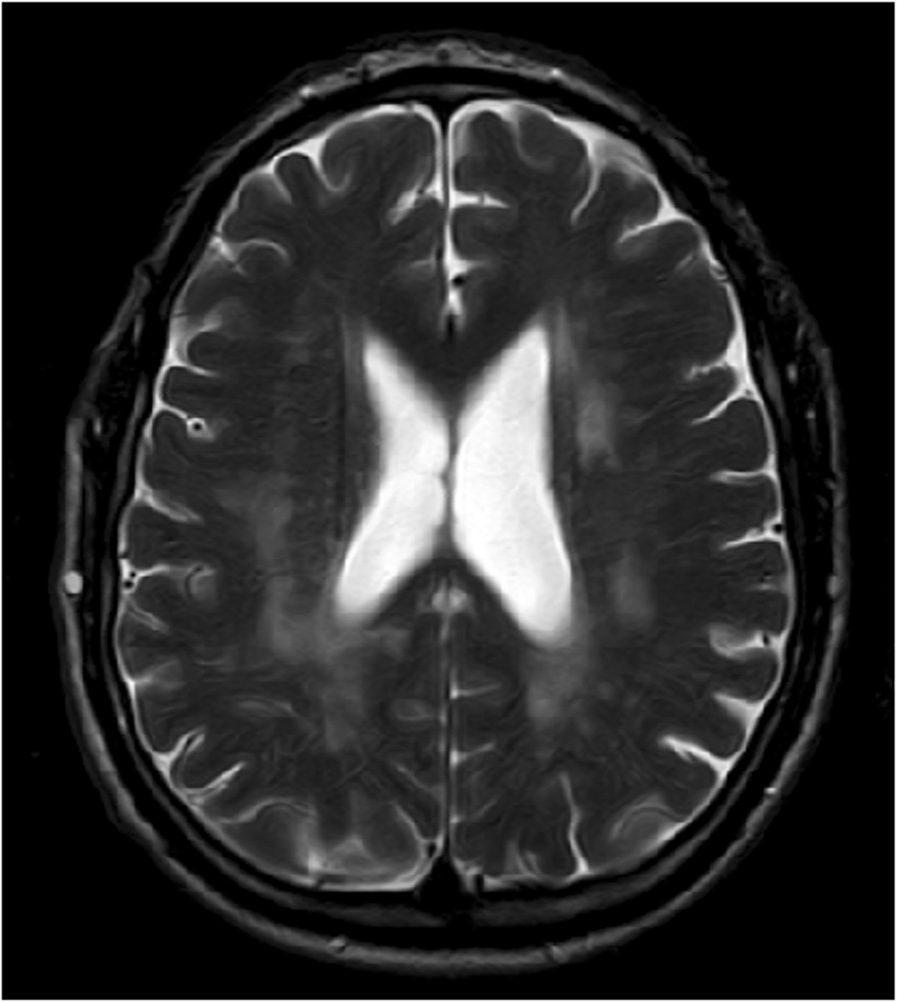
Central nervous system white matter lesions. 45-year-old male. T2 Brain MR image showed widespread, punctuated and confluent white matter lesions from periventricular to subcortical spaces. These lesions are associated with severe axonal injury (gliosis).
